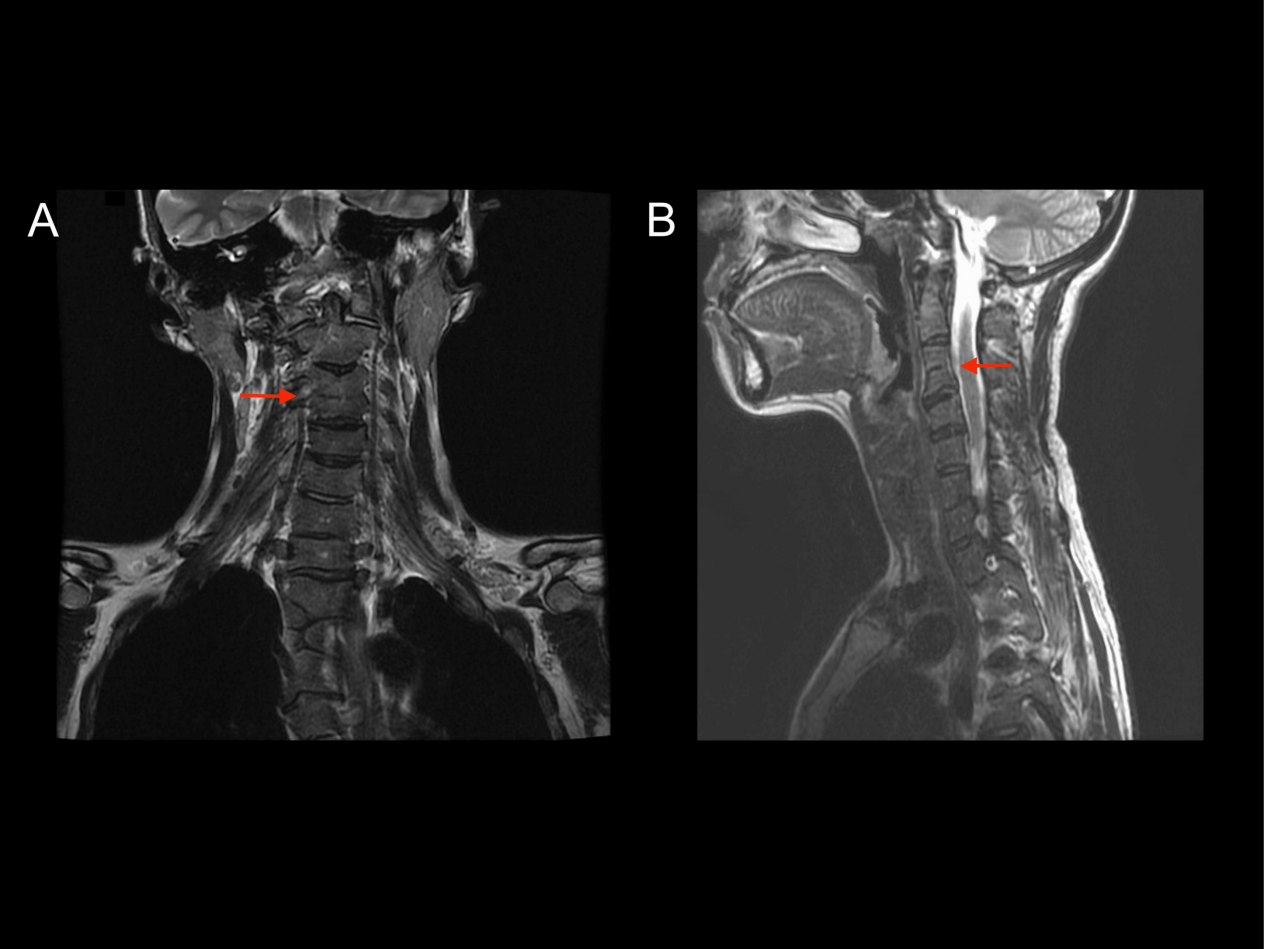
T2-weighted magnetic resonance imaging of the cervical spine and upper thoracic spine Cervical C3–4 fusion was noted (red arrow). The C4/C5 and C5/C6 discs are protruded.
